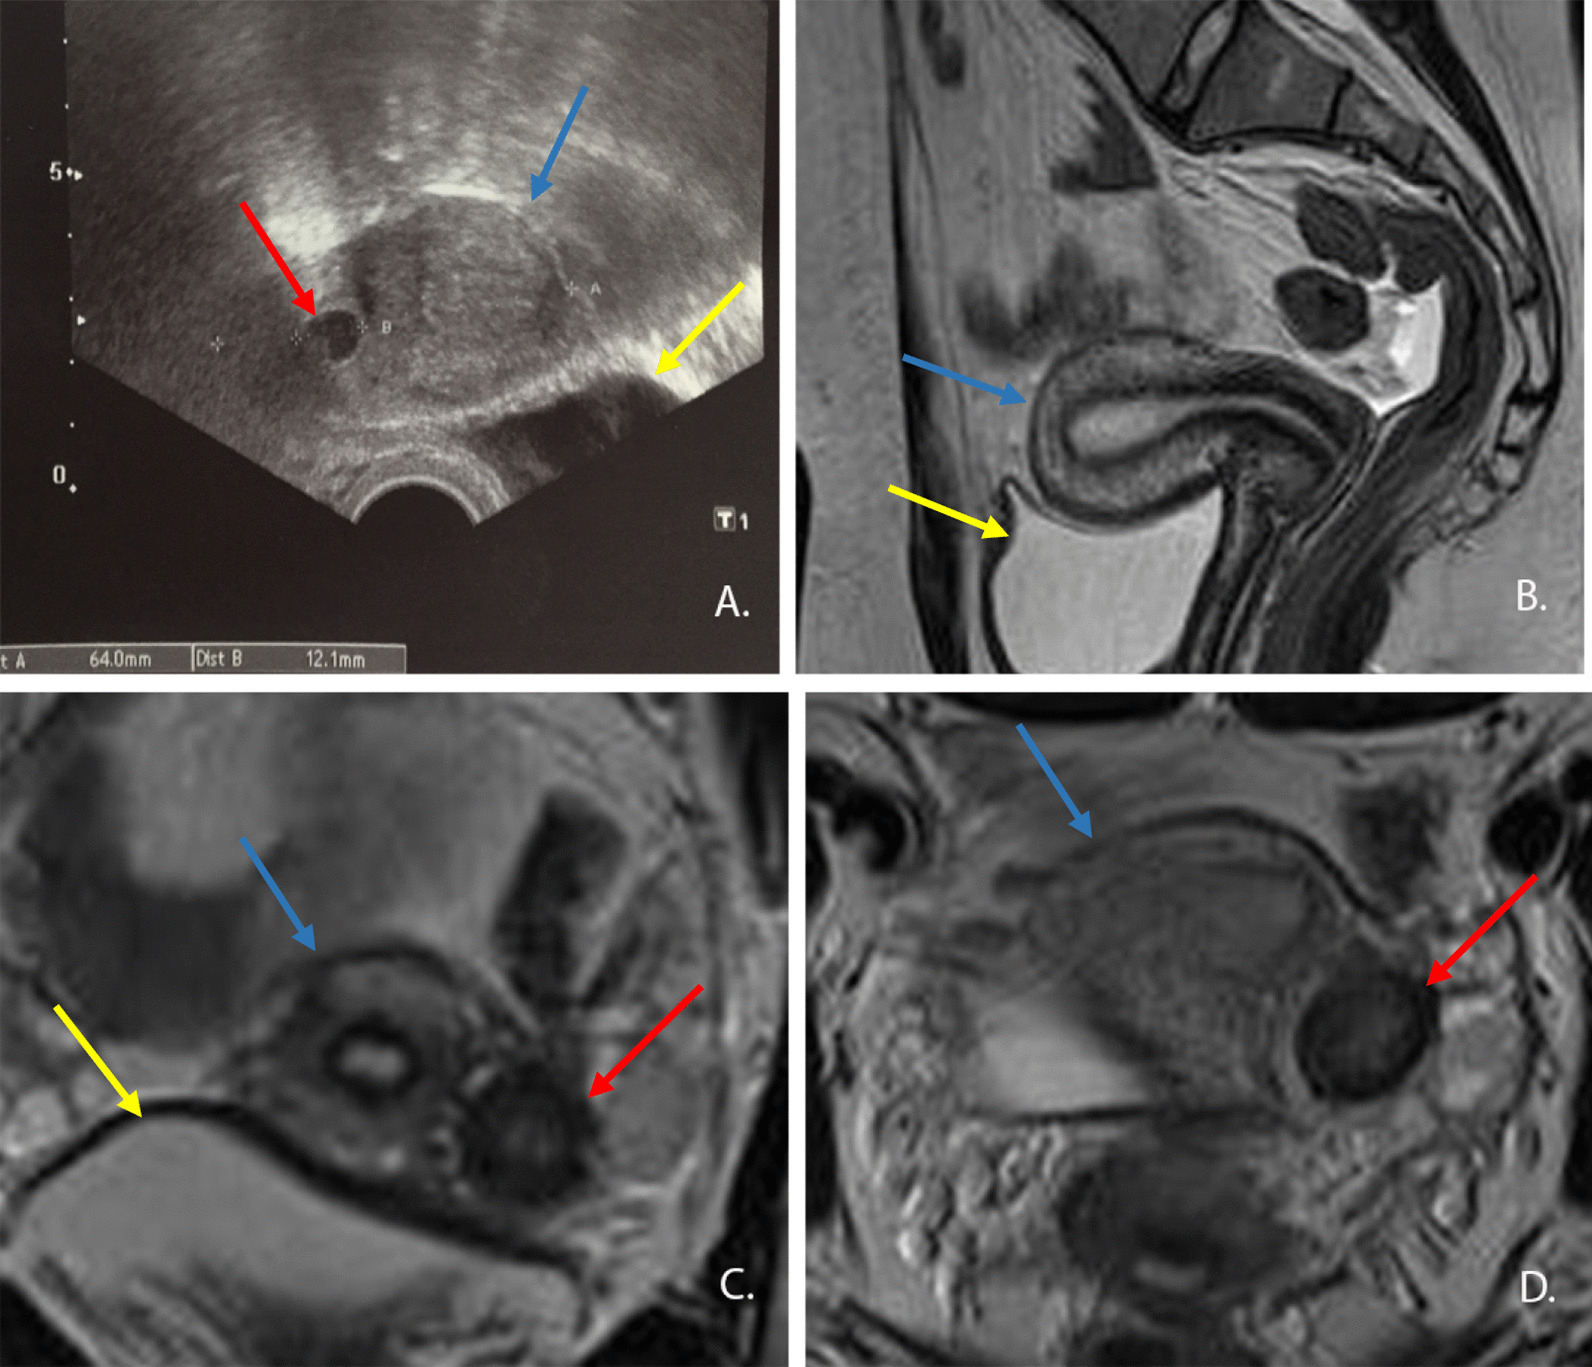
a Transvaginal ultrasound in the transverse plane (red arrows mark the rudimentary uterine horn, blue arrow the normal uterus and yellow arrow the bladder). b Magnetic resonance tomography (MRT) image in the sagittal plane. c MRT image in the transverse plane. d MRT image in the frontal plane
